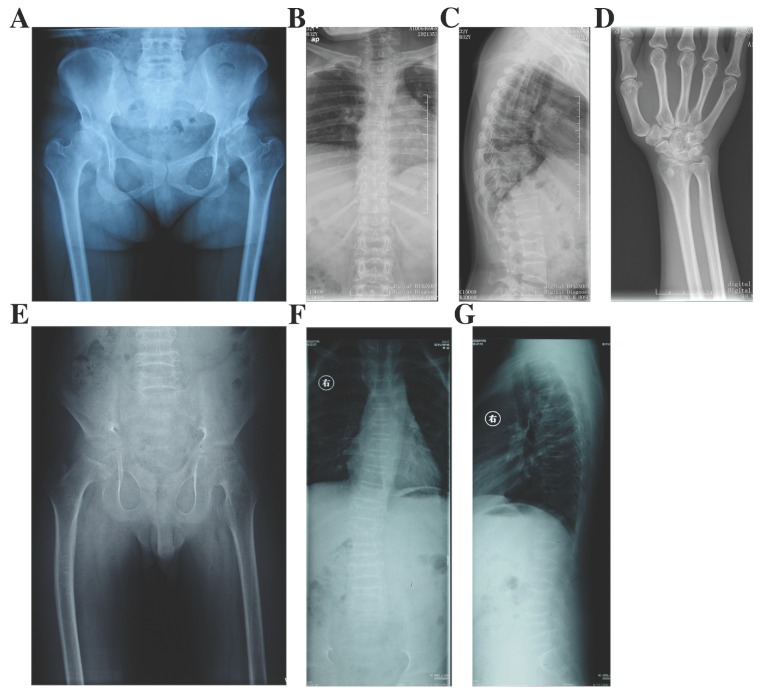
Radiographs of the probands with EDMMD and Kniest dysplasia. A-D: X-rays of pelvis (A), spine (B and C) and hands (D) of the proband in Family 9 with EDMMD. Osteoarthritis, coxa vara, shortening femoral necks and sclerosis of acetabulums were obvious in pelvis. Biconcave deformity, rough edges of vertebral body and narrow intervertebral disc spaces were significant in spine. Narrowing of the metacarpophalangeal and interphalangeal joint spaces and expansion of metaphyses were showed in hands and long tubular bones. E-G: Radiographs of pelvis (E) and spine (F and G) of the proband in Family 10 with Kniest dysplasia. Irregular shapes of femur heads, narrow joint space of bilateral hips, flat acetabular roof, coxa vara, severe multiple platyspondyly and slight scoliosis were showed.
